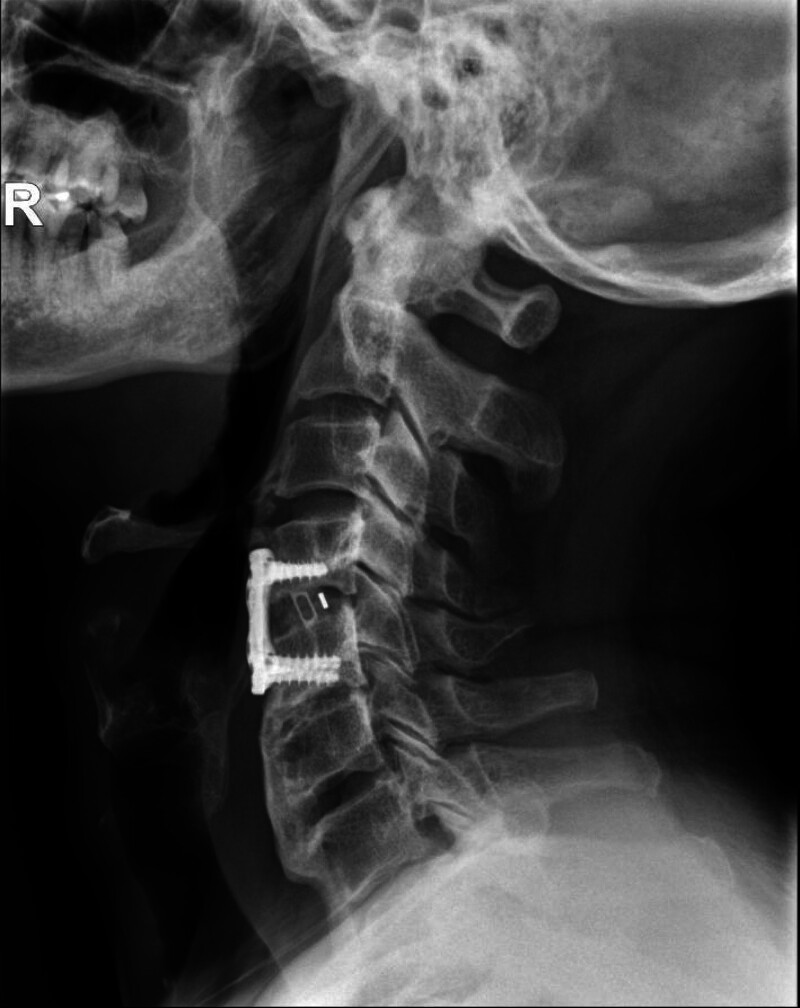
Lateral cervical X-ray at 3-year follow-up demonstrating partial regrowth of osteophytes along the anterior margins of C4 and C5.
